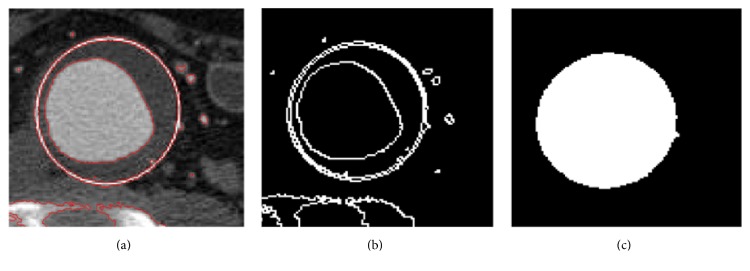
Processing steps required to calculate vessel area, shown for an example measurement at aneurysm sac level. The reconstructed CT images are segmented using the active contour algorithm (a), setting a seed point, and defining two ROIs in between which the vessel wall is found (b and c).
